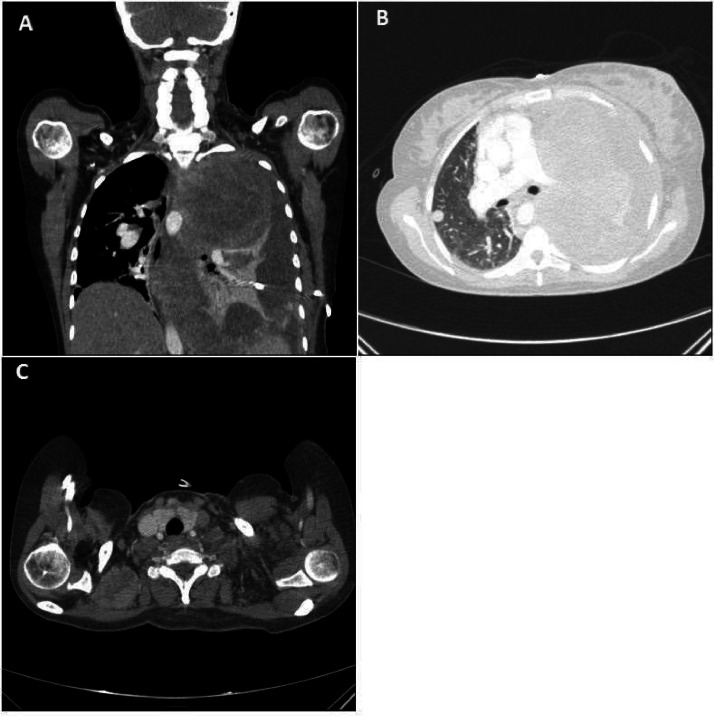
(A) Chest CT scan with IV contrast in coronal view. (B) Evidence of a large heterogeneous lobulated enhancing mass is seen in the left side of the mediastinum with extension to the left upper and lower lobes. Spiral chest CT scan in axial view showed a round pulmonary nodule in the lateral aspect of the right upper lobe. (C) Neck CT Scan Axial view showed heterogeneously enhancing soft tissue density lymph node in the supraclavicular region.
